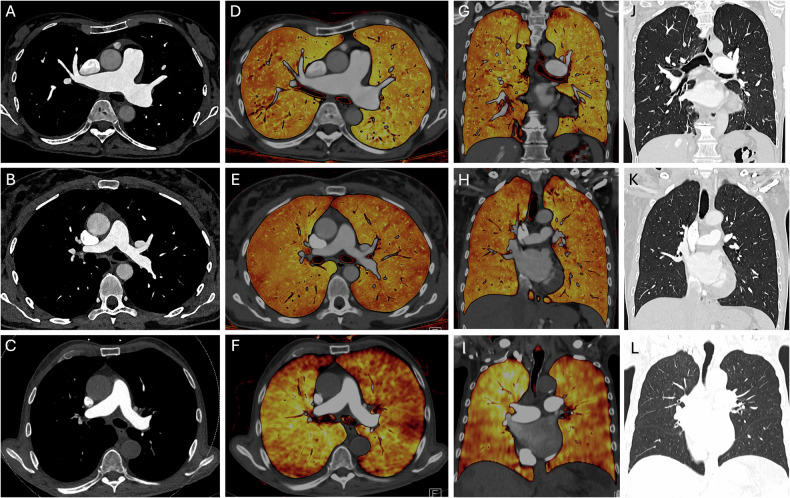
Exclusion of pulmonary embolism. Axial images of UHR-PCD-CTPA at IQ50 (A) and IQ25 (B) vs. EID-CTPA (C) with corresponding axial (D–F) and coronal (G–I) iodine maps, as well as coronal slices of the corresponding lung window (J–L). Note the excellent homogeneity of iodine maps of PCD-CTPA in UHR mode, irrespective of IQ level, while EID-CTPA iodine maps show some inhomogeneous spots that are not explained by underlying parenchymal pathologies like emphysema (see corresponding coronal lung window, L). CTPA, CT pulmonary angiography; EID, energy-integrating detector; IQ, image quality index; PCD, photon-counting detector; UHR, ultra-high resolution
